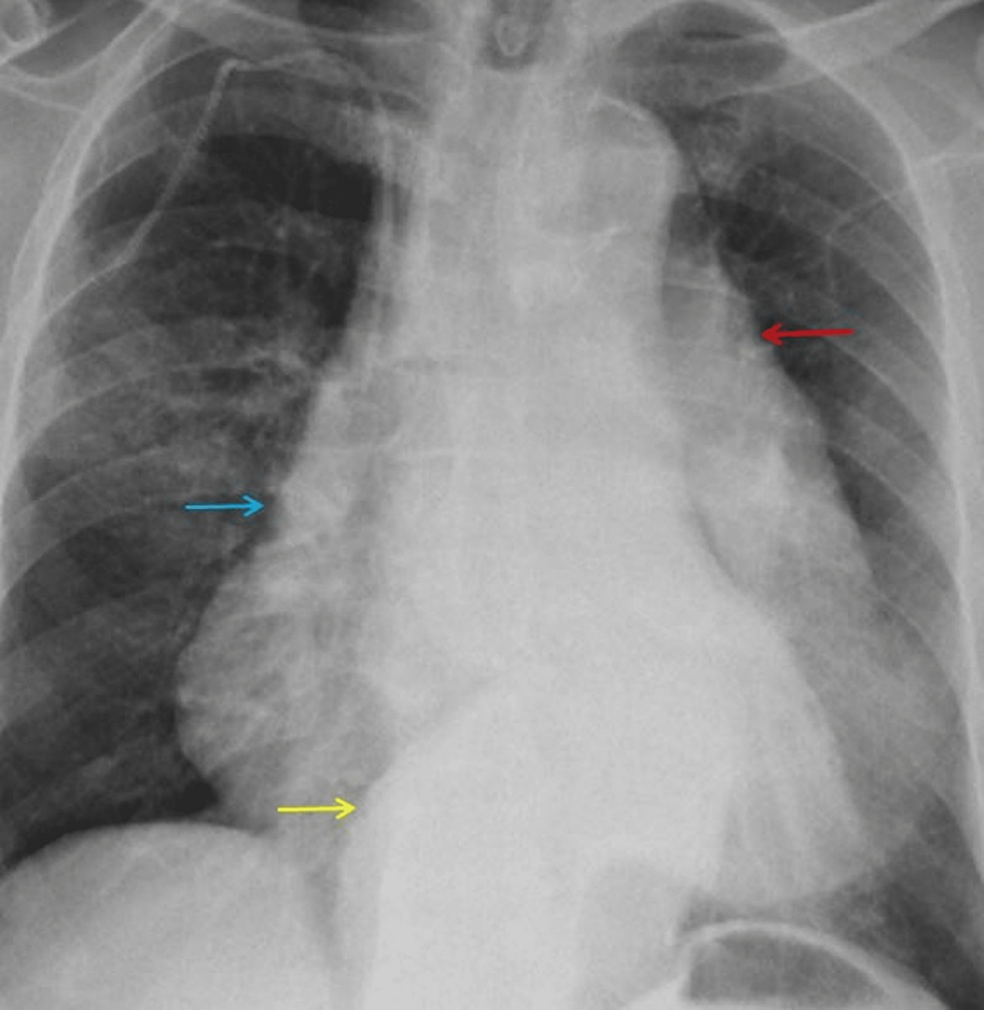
X-ray of the chest X-ray chest showing widened mediastinum (blue arrow), aortic aneurysm (yellow arrow), and obliteration of the aortic knuckle (red arrow).
